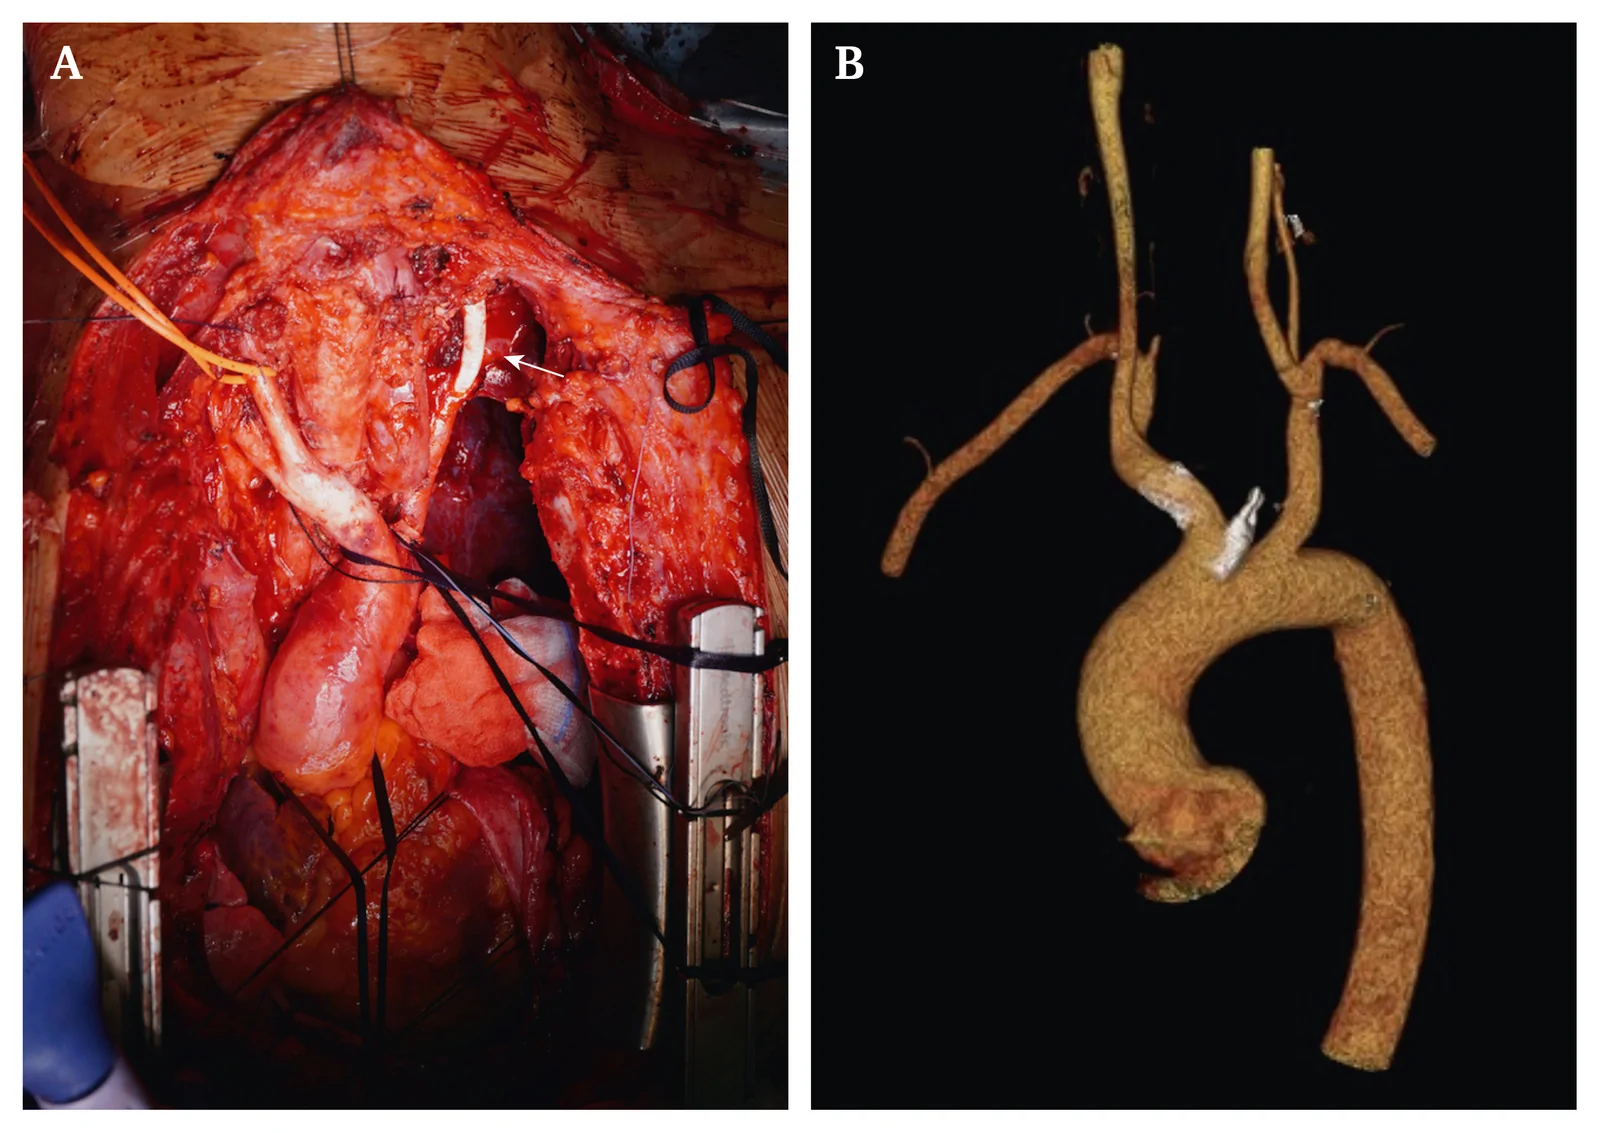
Final result after thymoma resection and left subclavian carotid bypass. (A) Intra-operative view showing the left subclavian artery to left common carotid artery prosthetic bypass (white arrow). (B) Three dimensional reconstruction from follow up computed tomography angiography.
